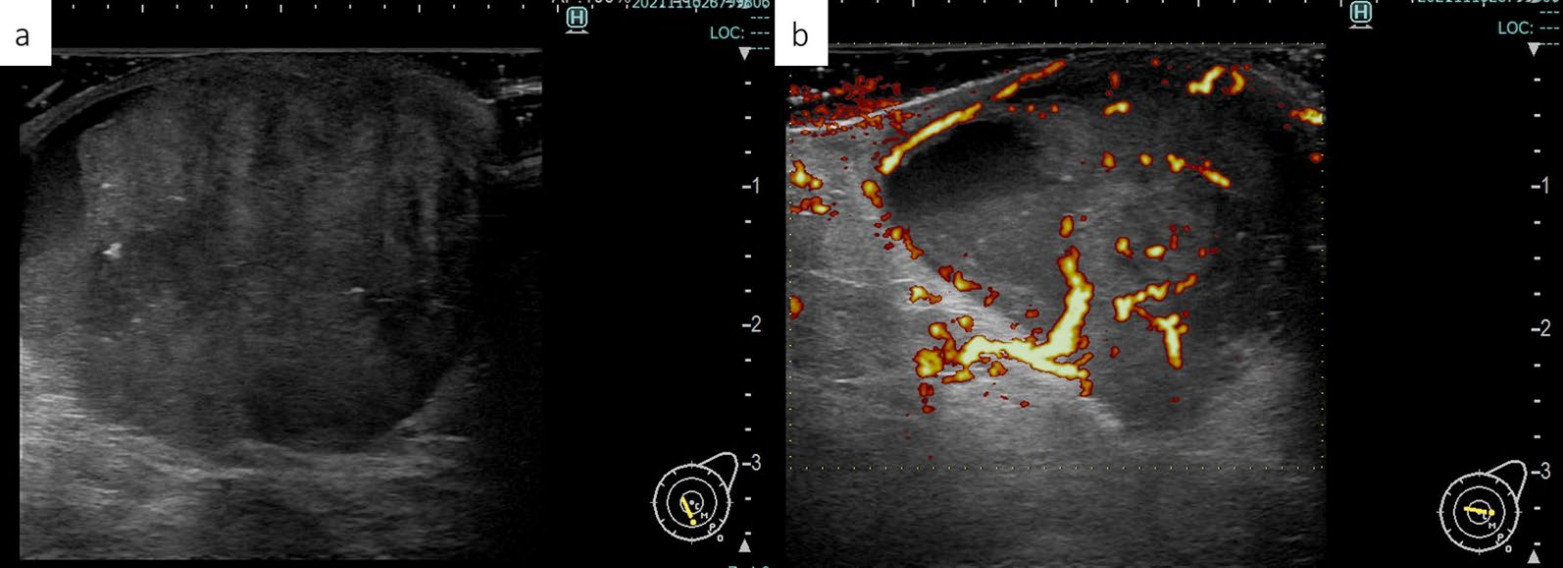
Breast ultrasound uncovered a hypoechoic mass measuring 39 × 31 mm with abundant blood flow in the left nipple area
